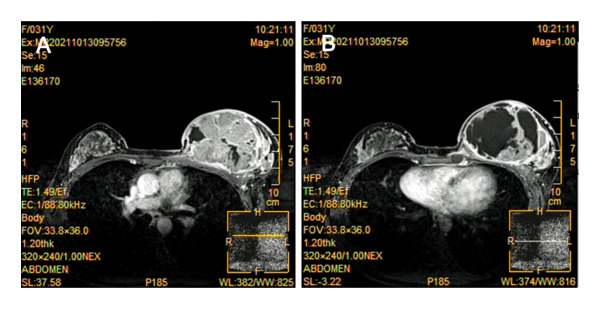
MRI demonstrated a significantly enlarged size of the left breast, demonstrating a breast mass and abnormal enhancement.
